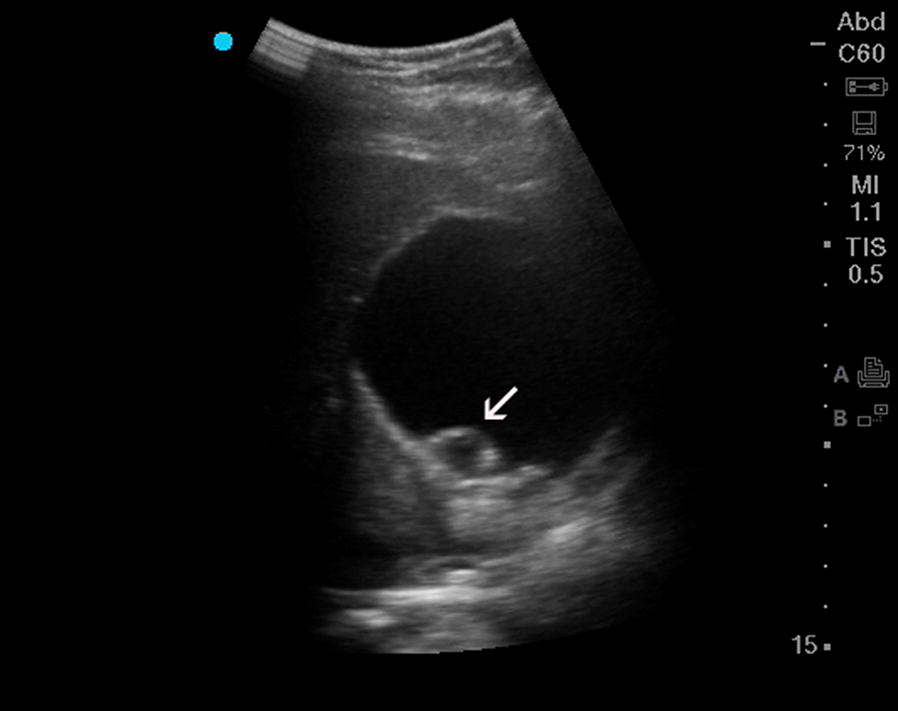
Ultrasound image of an *Echinococcus* cyst. Note the separation of the wall from the cyst (arrow) can be visualized. According to the WHO classification system, this cyst would be considered a type CE2 active cyst [3]
